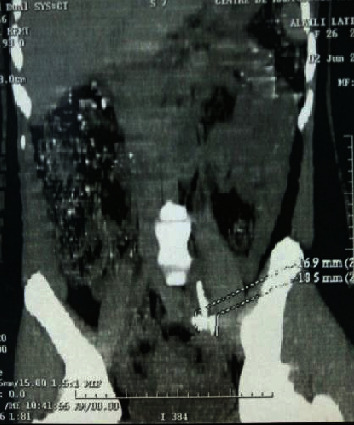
CT scan sagittal objective retroperitoneal localization of the IUD.
